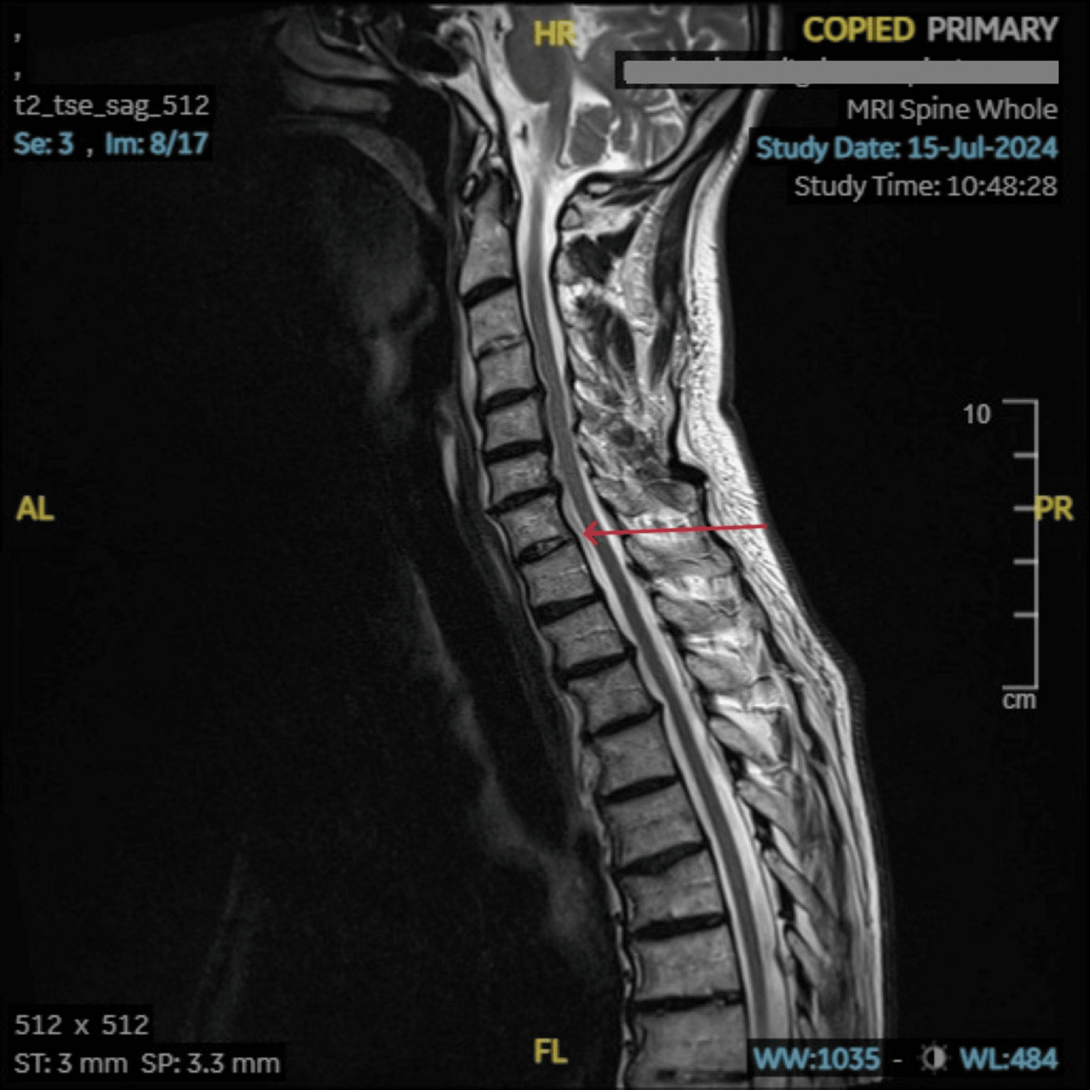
MRI scan of the spine A minimally displaced fracture involving the left transverse process of C4 with involvement of the left transverse foramina is seen. This is marked with a red arrow.
